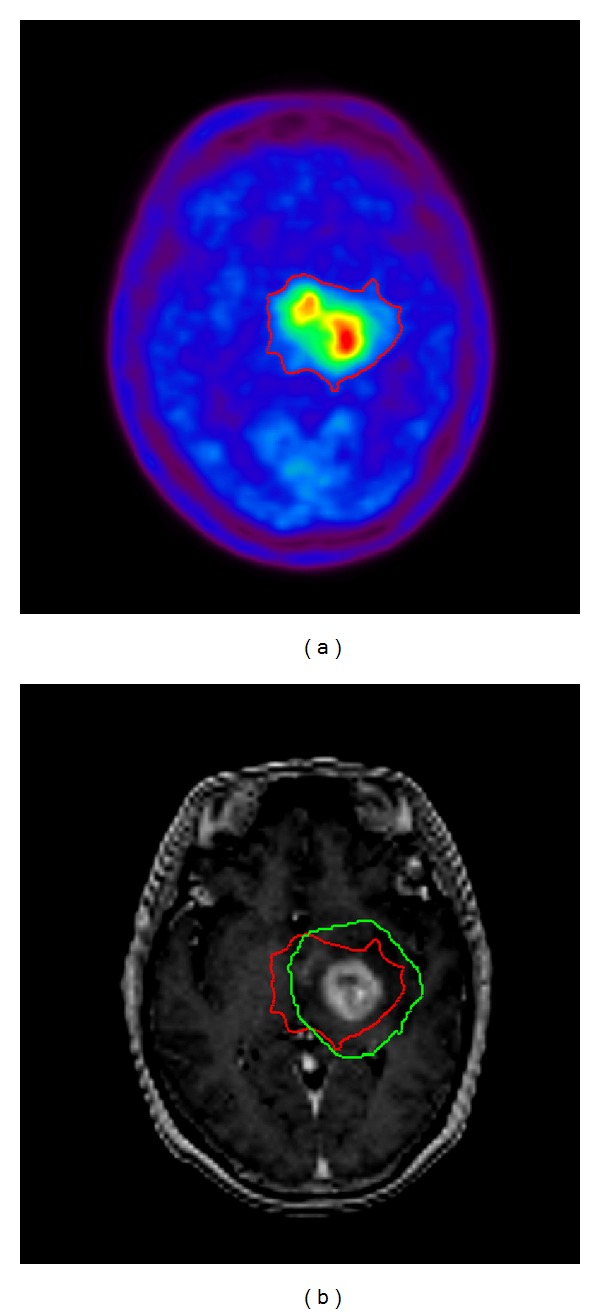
An example of the targets planned for a Hypo-IMRT procedure. (a) ^11^C-Methionine positron emission tomography (MET-PET). (b) Contrast-enhanced T1-weighted magnetic resonance imaging (MRI). Gross tumor volume (GTV) was the area of enhanced lesion on MRI, including MET-PET avid region. Clinical target volume (CTV) was the area with 5 mm margin surrounding the GTV. Both MET-PET moderate region (red line) and the area with 15 mm margin surrounding the CTV (green line) were included in planning target volume. The final determination of target delineation was obtained by consensus among three observers.
